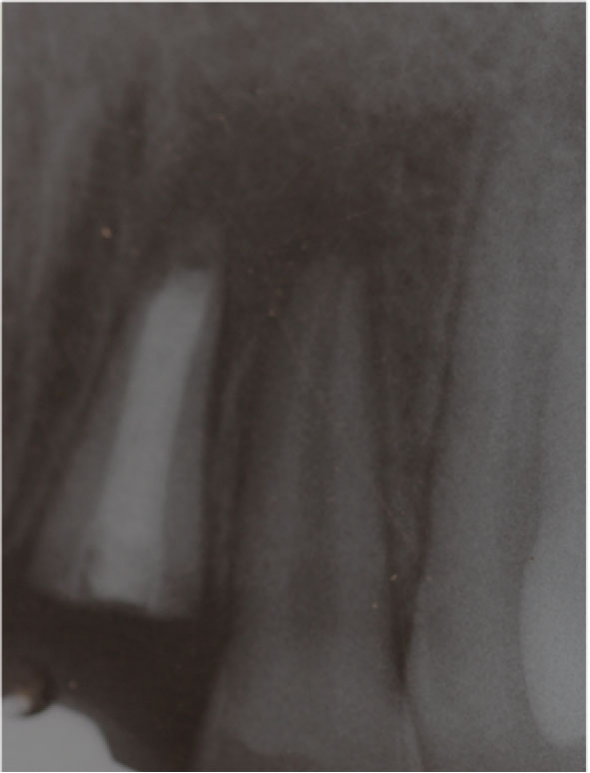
Periapical radiograph revealing large radiolucent area associated with the apex of tooth #21.
